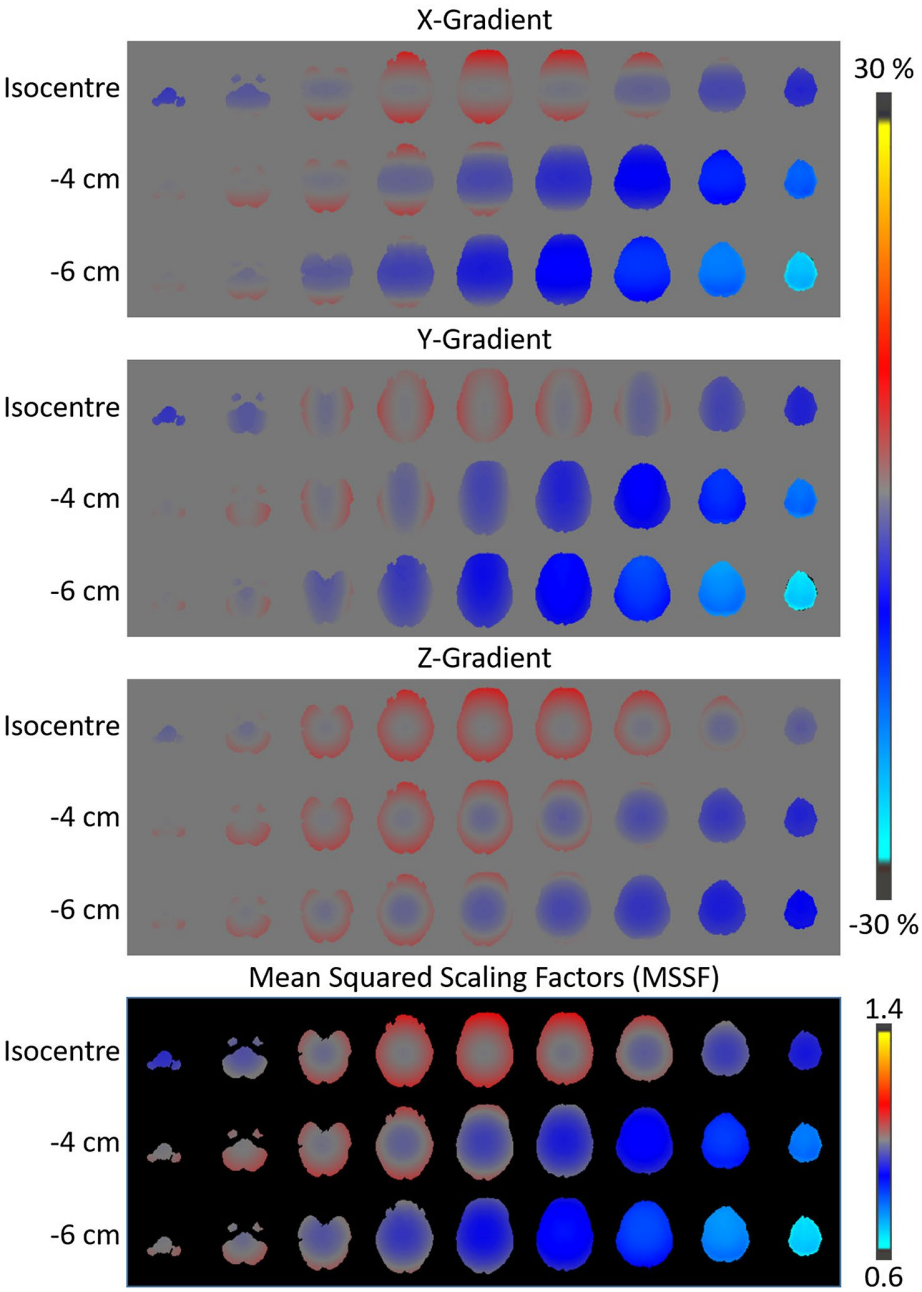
Volunteers were scanned using a diffusion MRI protocol on a 300 mT/m Connectom scanner with different bed translations. *X*, *Y* and *Z* gradient nonlinearities from one of the subjects is presented here for a subset of bed translations. As the volunteers were moved further into the scanner, the gradient nonlinearity effects became more severe and reached nearly −30% of nominal value. The mean of the square of gradient scaling factors (MSSF) due to gradient nonlinearity from the same volunteer is provided at the bottom of the figure and shows significant MSSF variations across bed positions
